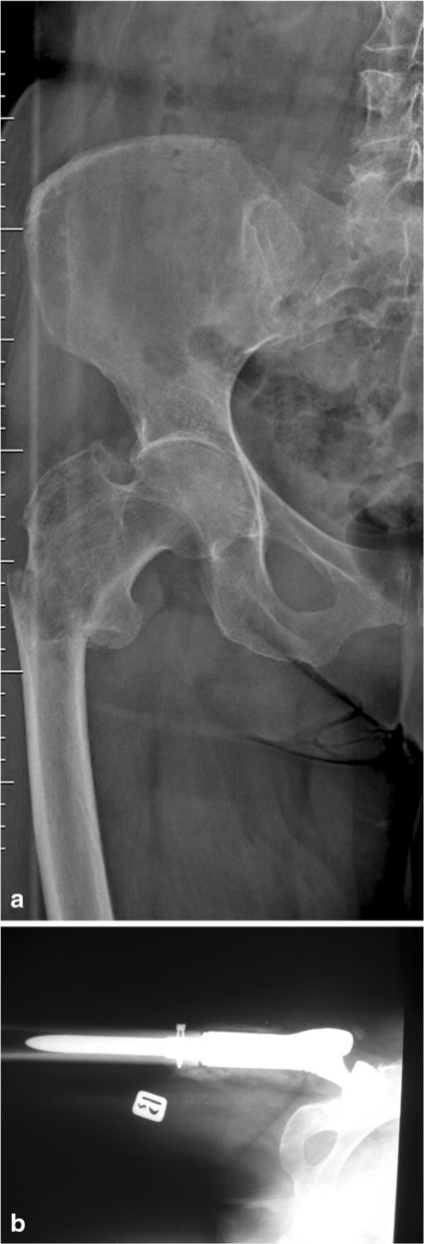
Radiographs of proximal femur destruction by metastatic renal cancer (a) and modular endoprosthetic replacement (b)
